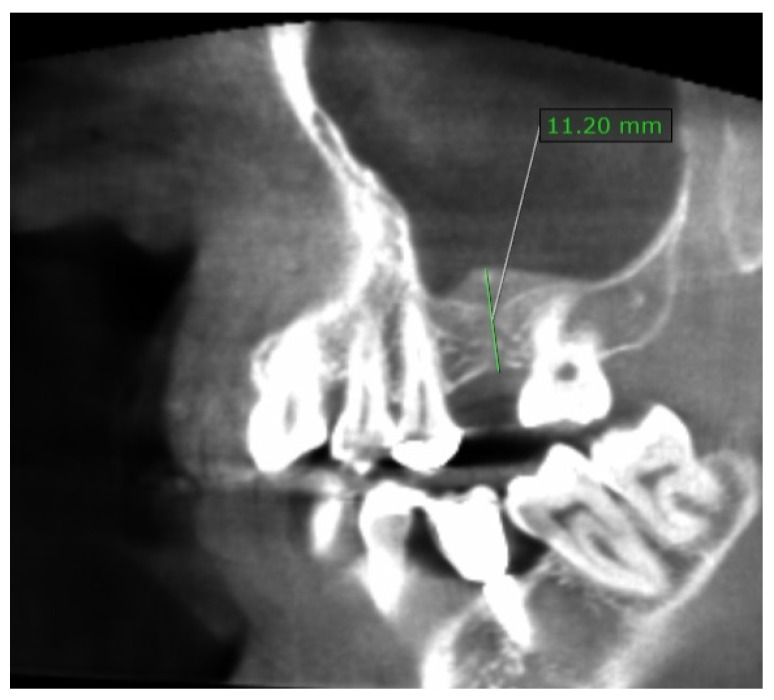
The section of the CBCT showing a residual height of 11.2 mm after 6 months from the sinus lift, meaning an earning bone height of 7.6 mm. The bone seems to be of good quality for dental implant placement.
